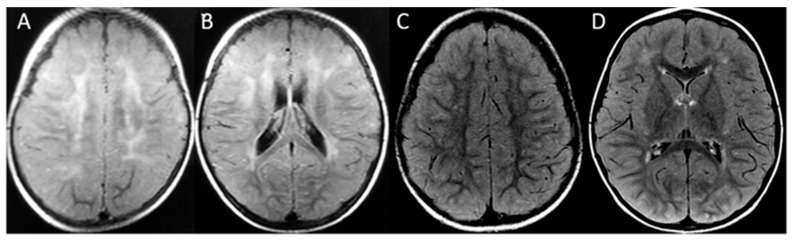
Patient 2. First MRI at 14 months old, axial FLAIR (A,B) demonstrates the frontoparietal patchy and asymmetric distribution of signal hyperintensity at the cerebral white matter. The second MRI at age 4 (C,D) shows a marked reduction in leukopathy. No atrophy is seen at any time.
